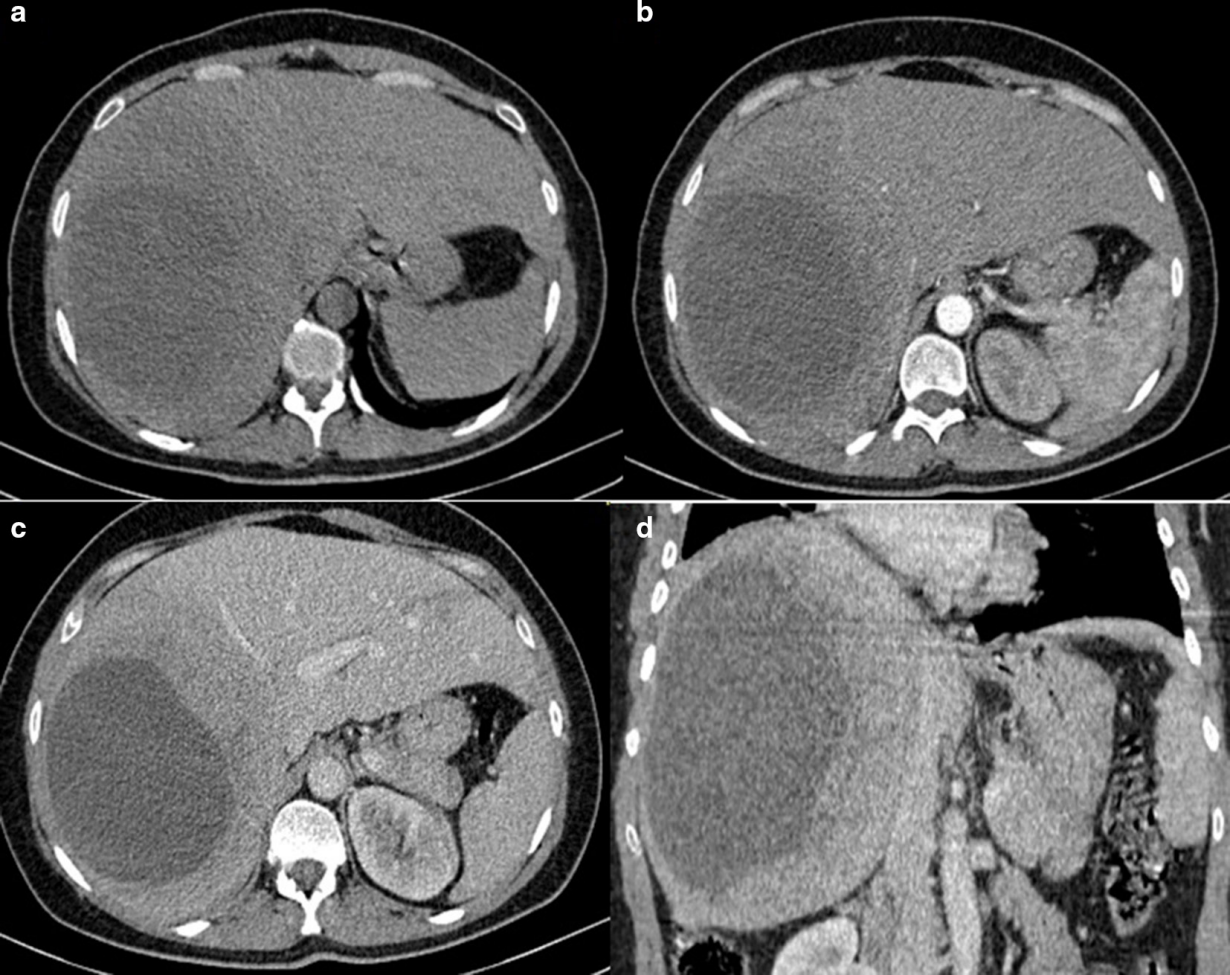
(A) Non-contrast CT scan showing a large hypodense lesion with thick wall in the right lobe of the liver (arrow). (B) Contrast-enhanced scan on arterial phase shows irregular peripheral enhancement (arrow).(C-D) Contrast-enhanced scan on portal phase shows no significant change (arrow).
